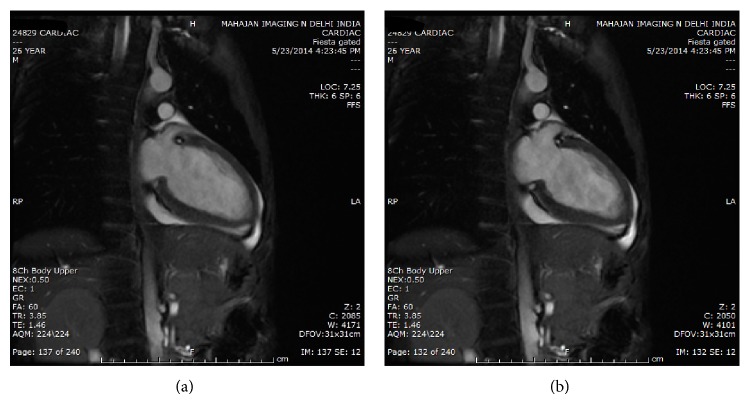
(a) Cardiac MRI of patient. (b) Coronal view cardiac MRI of patient 1.
